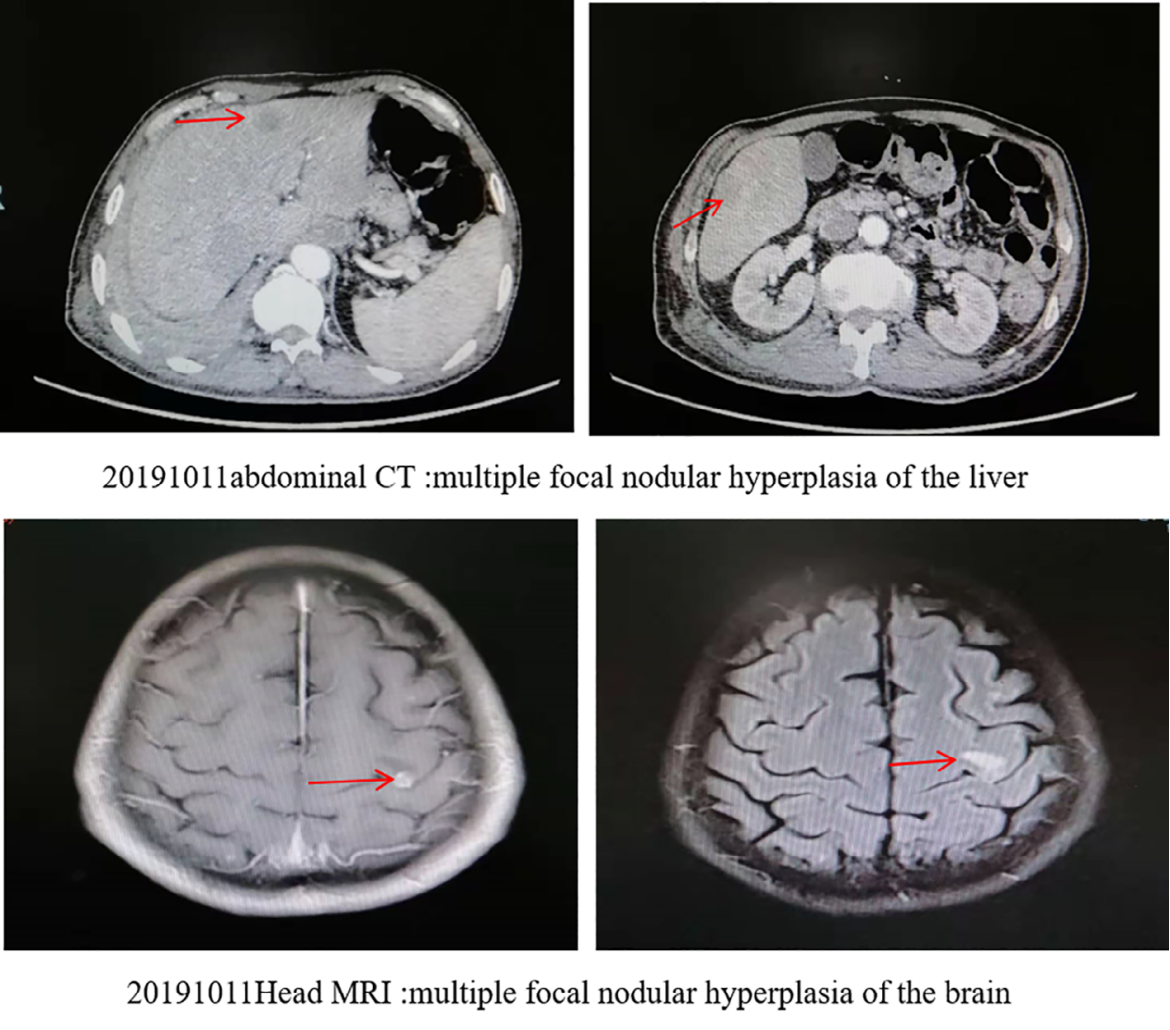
The CT scan findings two months after treatment with osimertinib and afatinib. The red arrow indicates liver and brain metastases.
